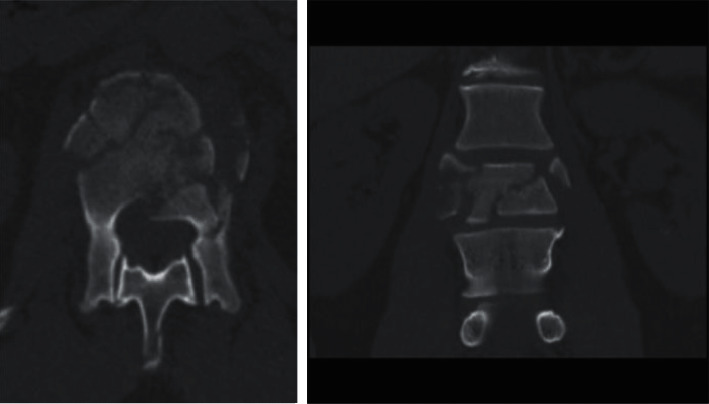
Axial and coronal CT scan of L1 burst fracture after a snowmobile accident.
